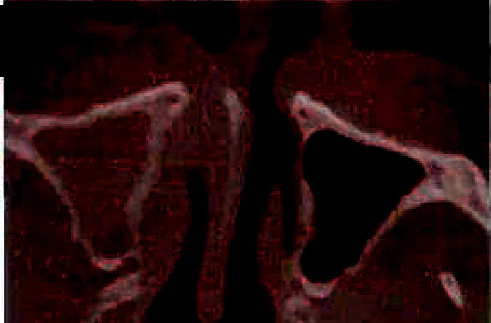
CBCT image of apical periodontitis by the threshold segmentation algorithm.
